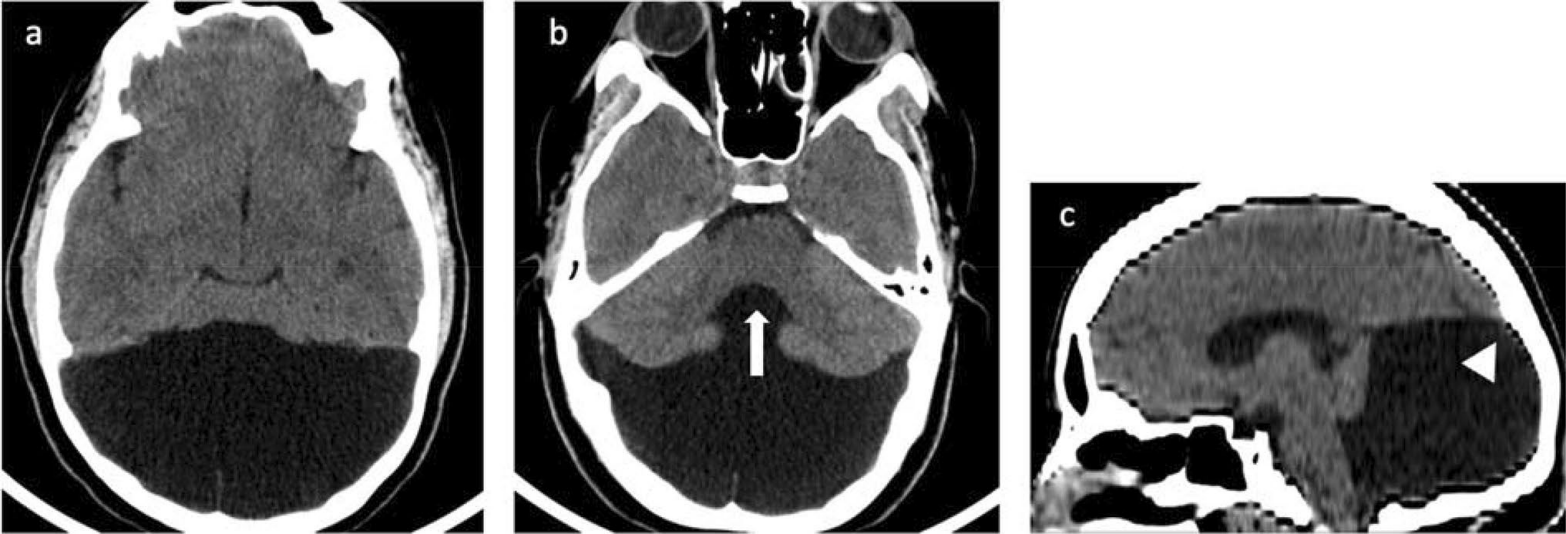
Axial non-enhanced computed tomography (NECT) scans (a, b) and sagittal MPR (c) in 12-year-old boy with Dandy–Walker malformation NECT shows all the main features of DWM: a large posterior fossa cyst, hypoplastic cerebellar hemispheres, and absence of the vermis (white arrow). Sagittal T1W image (c) show a massively dilated fourth ventricle, expanded posterior fossa, and elevated torcular herophili and transverse sinuses (arrowhead) and hypoplastic cerebellum
